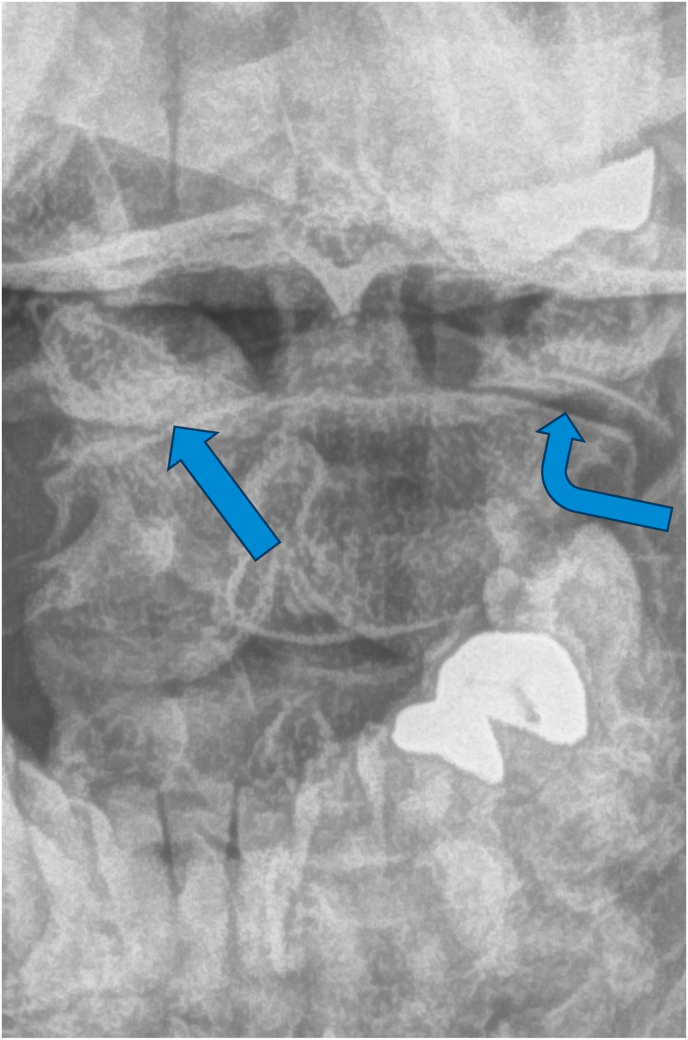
X-ray from a patient with right suboccipital pain demonstrating severe arthrosis of the right C1-2 joint (straight arrow) with a well-preserved joint on the left side (curved arrow).
